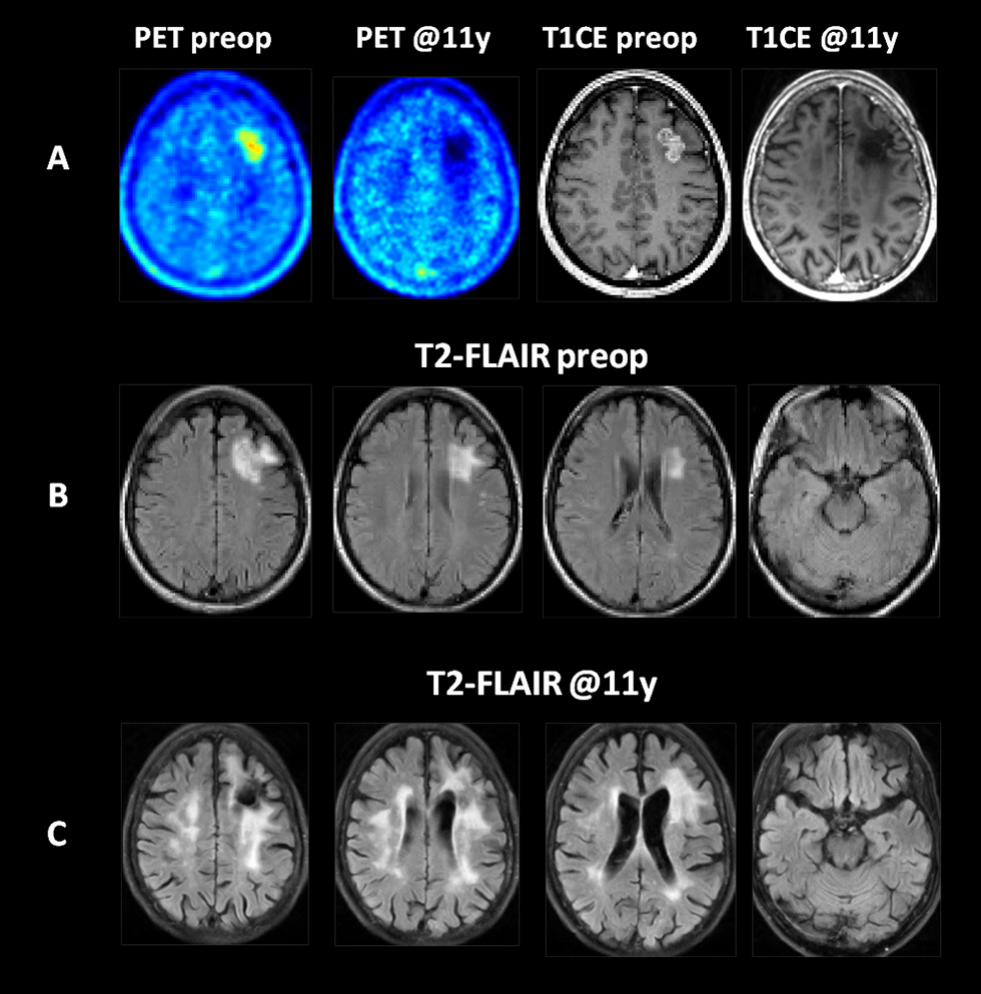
Imaging findings at initial diagnosis and at 11 years of follow-up in a patient with a left frontal NSCLC (adenocarcinoma) brain metastasis treated by resection and whole brain radiotherapy. (A) The T1-weighted contrast enhanced MR (T1CE) and FET PET (PET) images revealed a contrast enhancing, PET-positive metastatic tumor with no evidence of recurrence in the brain or resection cavity during follow up. (B) The T2-FLAIR images at initial diagnosis. (C) At follow-up, extensive areas of T2-FLAIR hyperintensities developed in the white matter of the bilateral dorsal frontal and parietal lobes. All images are shown after elastic registration to the MNI-152 standard brain template.
